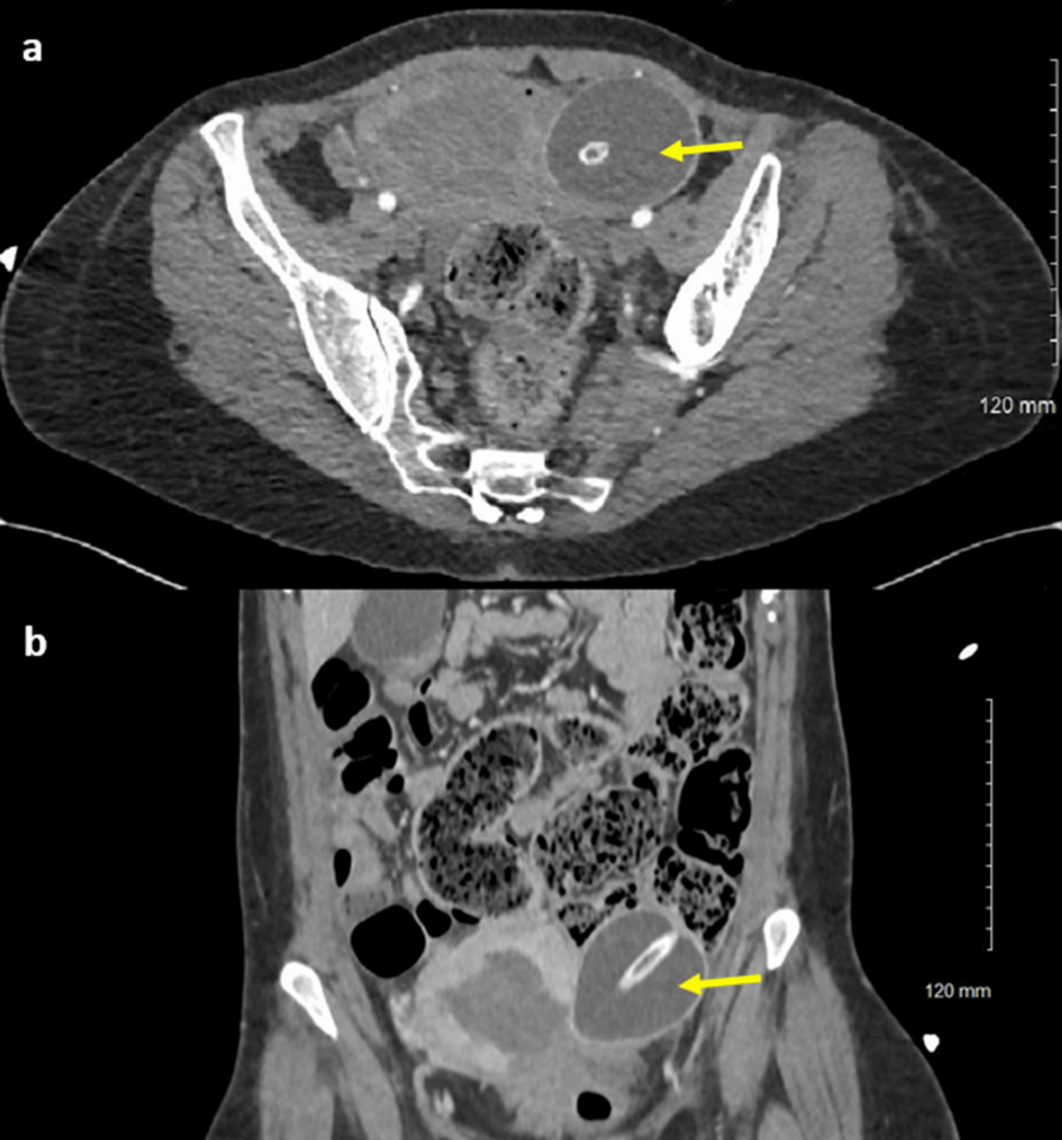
Axial (a) and coronal (b) CT with contrast images taken before transfer to theatre The images show a large defect in the uterine wall with appearances suggestive of a perforated uterine body into the pelvis (yellow arrow).
